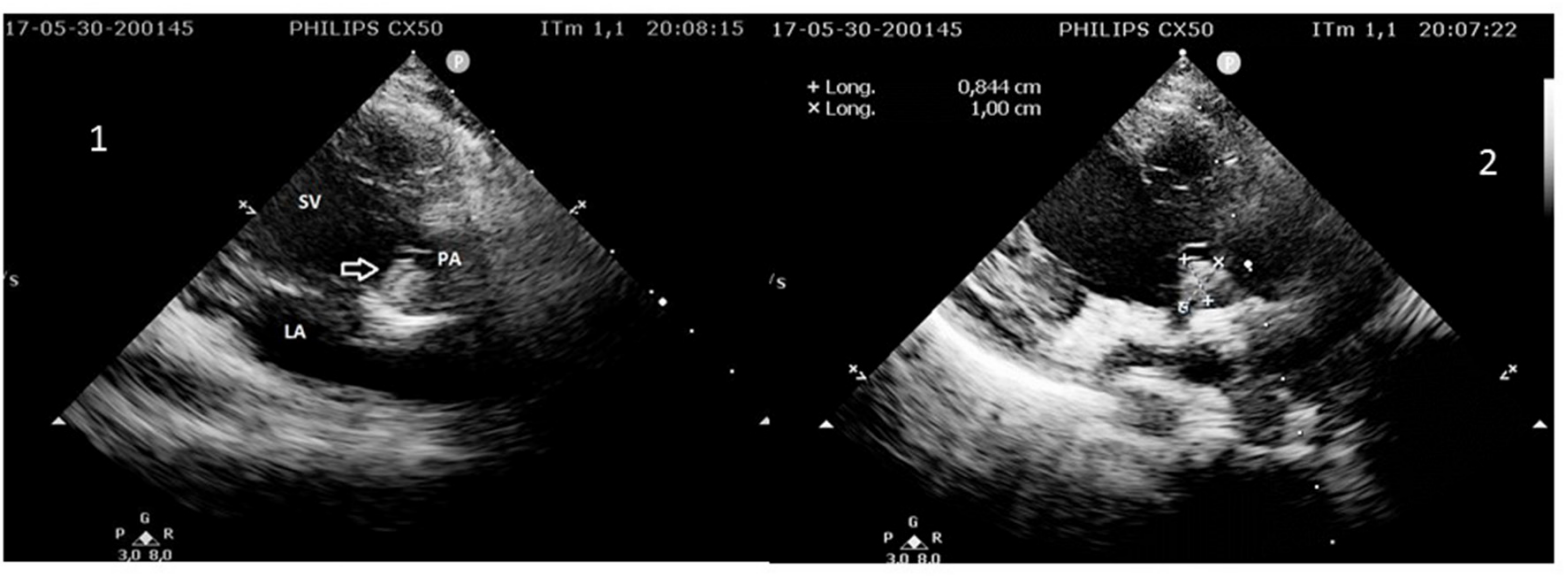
1. 2D echocardiography showing the mobile thrombus (arrow) inside the pulmonary artery (PA) trunk arising from the single ventricle (SV). LA, left atrium. 2. Measurement of the thrombus in the pulmonary artery (PA) trunk arising from the single ventricle (SV).
